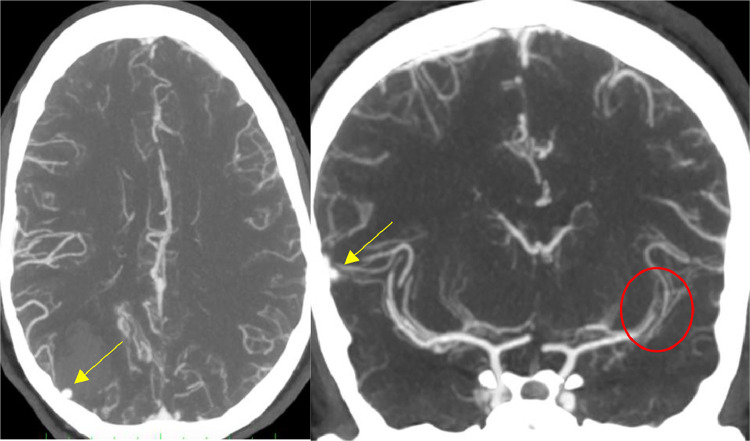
CTA of the brain, in axial (left) and coronal reconstruction (right), showing a saccular pseudoaneurysm in the right parieto-occipital lobe (Yellow arrow), and occlusion of the M3 segment of the left middle cerebral artery (Red circle).
